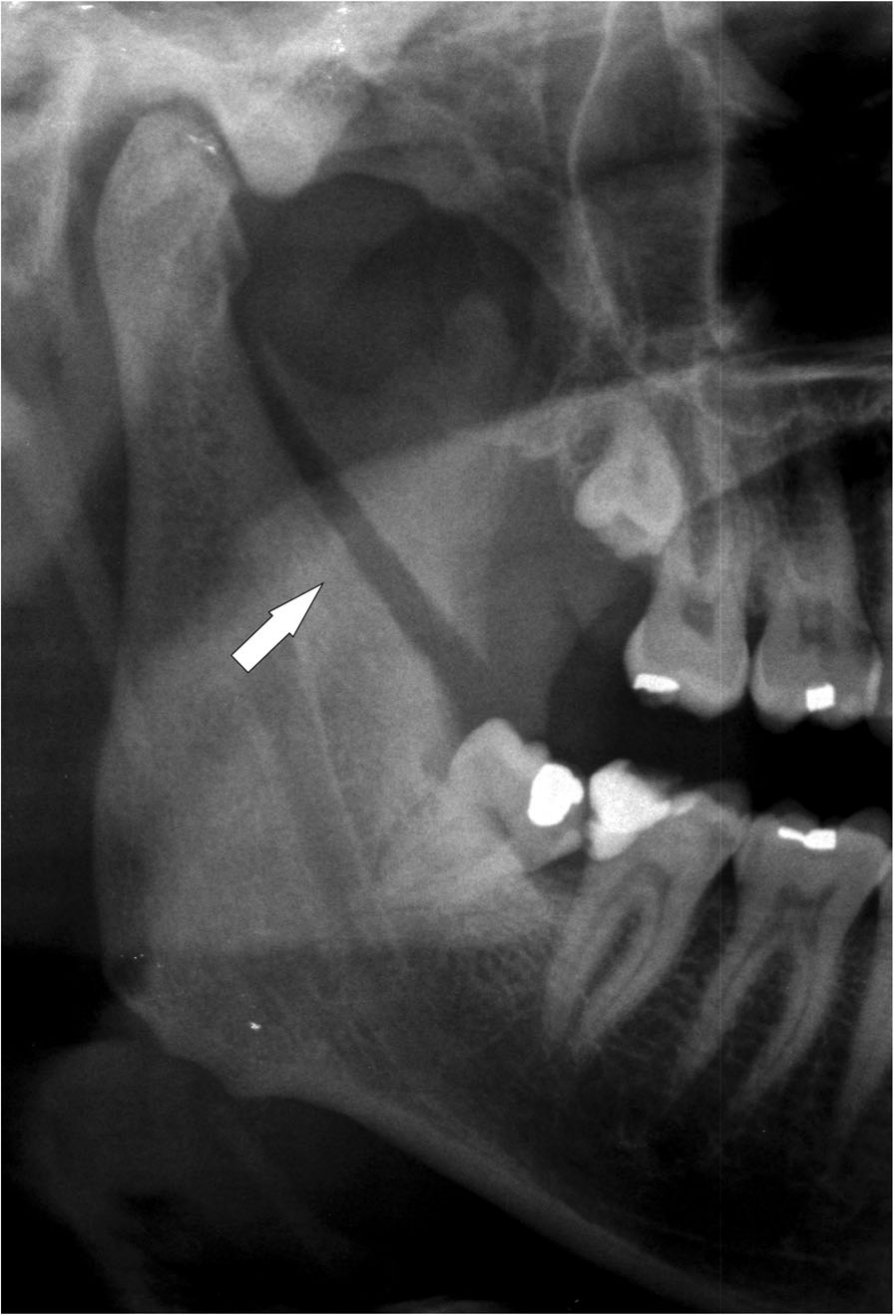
Coronoid process fracture lateral to the deepest central point of the sigmoid notch. Cropped panoramic radiograph showing a displaced fracture of the right coronoid process (arrow). The temporal muscle elevates the bone fragment upwards
